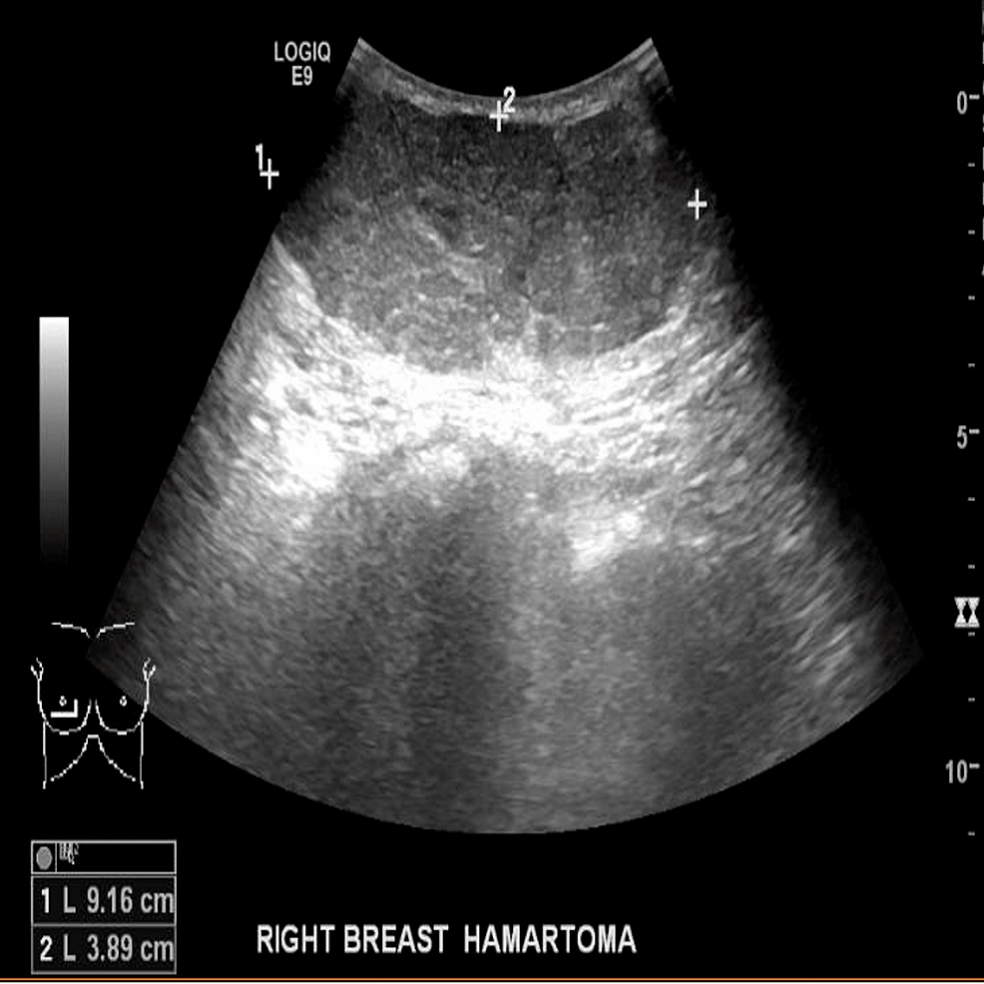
Right breast USS prior to pregnancy showing a U3 52 mm, heterogeneous hypoechoic lesion. USS, ultrasound scan
